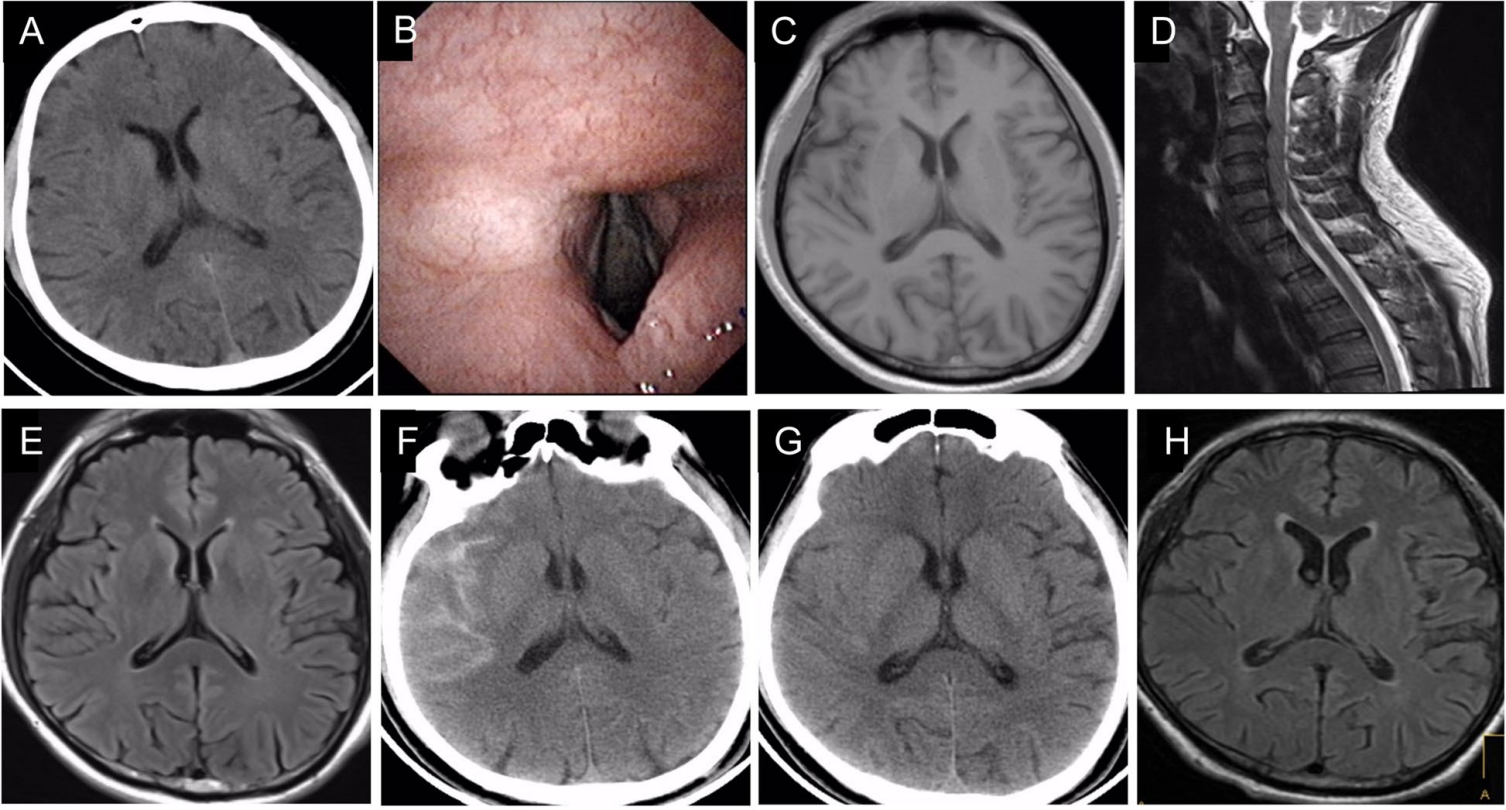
Brain computed tomography (CT) showed no obvious abnormality. Electronic laryngoscope showed slight hyperemia of the laryngeal mucosa. The findings were in accordance with changes in chronic laryngitis. Brain magnetic resonance imaging (MRI) on the second day after admission showed no obvious abnormalities. A Cervical spine MRI on the second day showed multiple cervical disc herniations. B Repeat brain MRI on the 4th day showed no obvious abnormality. C Head CT showed subarachnoid hemorrhage. D Repeat head CT showed that the subarachnoid hemorrhage has been absorbed after one month. E Repeat brain MRI on the 55th day showed no abnormality
